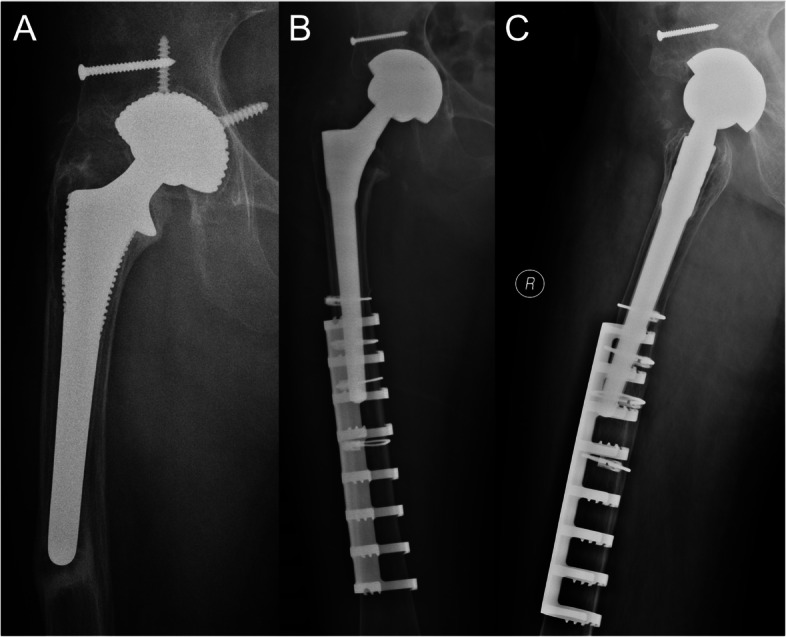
A 77-year-old female patient developed right aseptic loosening 15 years after primary hip arthroplasty. A Preoperative radiograph. B Immediately after surgery. The acetabulum and femur was revised, and a SSEC was inserted. C 10 years after surgery, the stem was stably fixed and there was no evident bone resorption around the proximal femur
